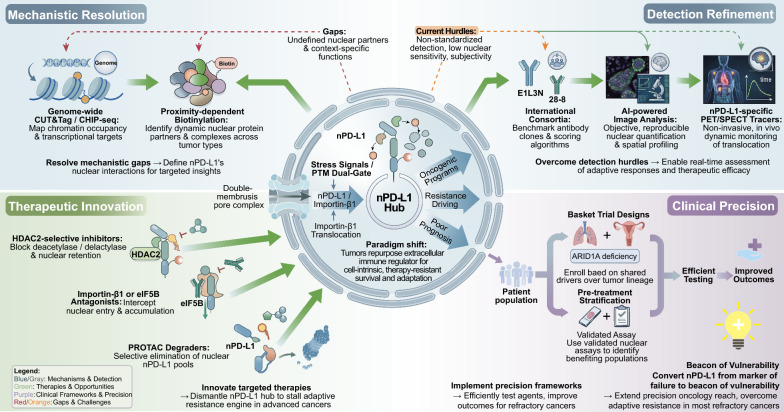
Precision targeting of nuclear PD-L1: future mechanistic, detection, and clinical directions. This mind-map summarizes current gaps and proposed strategies for exploiting nuclear PD-L1 (nPD-L1) as a therapeutic vulnerability. Mechanistic resolution approaches, including proximity labeling, CUT&Tag/ChIP-seq, and biotinylation-based methods, may help define nuclear interactors, chromatin occupancy, and tumor-type-specific complexes. Detection refinement strategies include standardized multiplex imaging, AI-assisted quantification, international benchmarking of antibodies, and the development of nPD-L1-specific PET/SPECT tracers for non-invasive dynamic monitoring. Therapeutic innovation may involve selective HDAC2 inhibitors, blockers of the importin-α/β1 pathway and eIF5B, and nuclear-specific PROTACs to dismantle the adaptive resistance hub. Clinical precision frameworks include biomarker-driven basket trial designs based on shared molecular features such as ARID1A deficiency, pre-treatment stratification, and validated nuclear assays to identify responsive populations. Two speculative but mechanistically grounded extensions are shown as dashed pathways: the intercellular spread of nuclear-import-competent exosomal PD-L1 within the tumor microenvironment, and the mitotic inheritance of PTM-primed nPD-L1, whereby daughter cells may bypass de novo modification requirements and rapidly reconstitute a resistance-competent nuclear program. Overall, the long-term goal is to shift nPD-L1 from a marker of therapeutic failure to a potential beacon of vulnerability in refractory cancers
